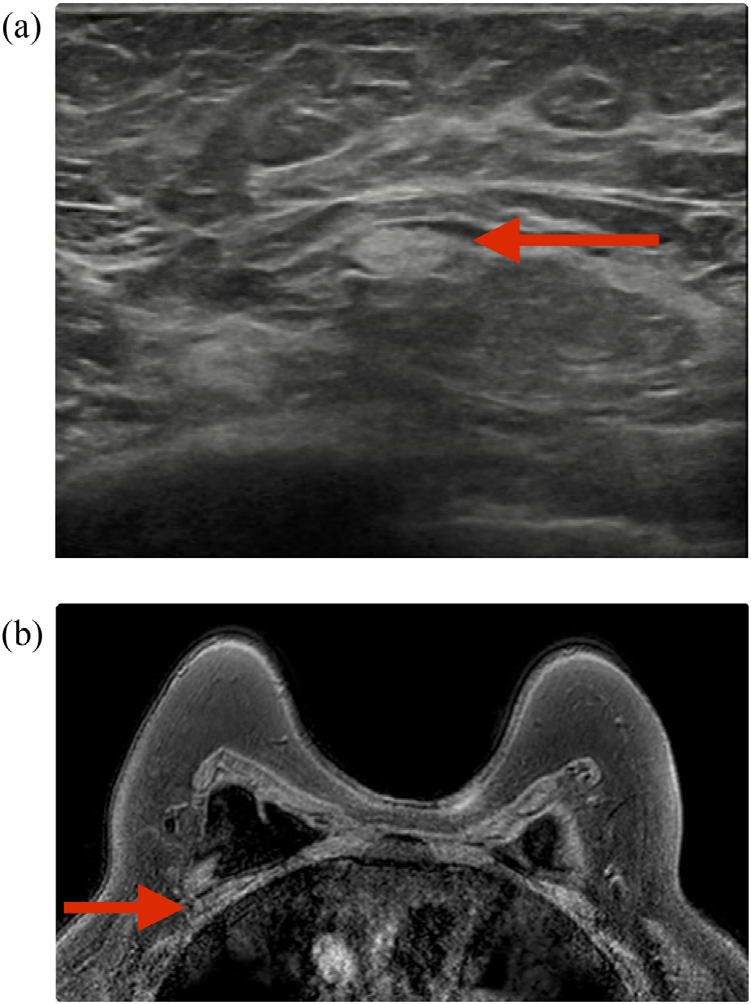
Imaging of axillary US and breast MRI exam of a patient with SLA.
